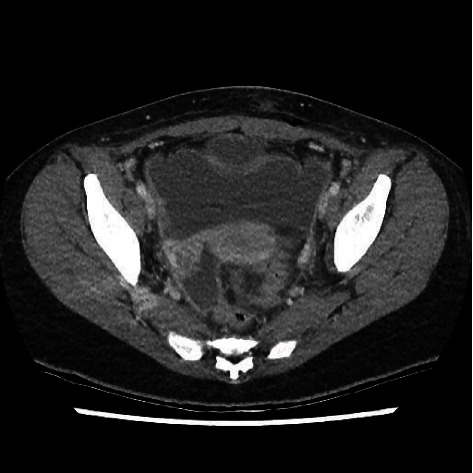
Enhanced abdominal CT scan five days after initial CT scan and first intervention showing increased amount of free pelvic fluid.
